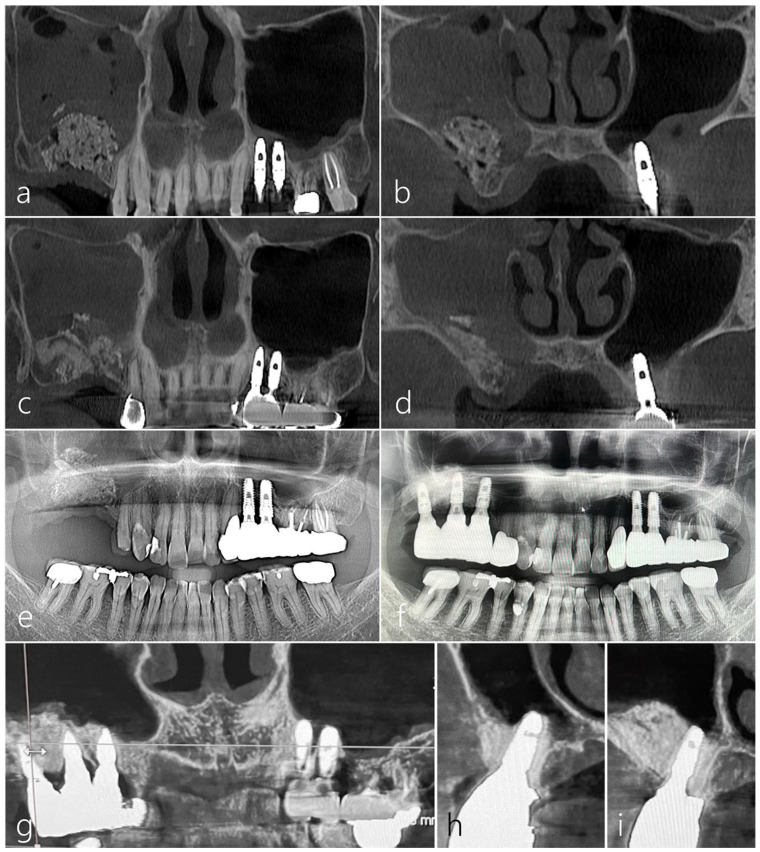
(a) The panoramic image of the CBCT taken immediately after surgery revealed an augmented bone graft in the right sinus. (b) On the coronal image of the CBCT, an augmented bone graft was observed in the buccal bone defect around the OAF site. (c) The panoramic image of CBCT taken 6 months later showed well-maintained augmented bone height. (d) On the coronal image of the CBCT taken 6 months later, some contraction of the bone graft area was observed, and the maxillary sinus was completely opacified. (e) Panoramic radiography conducted 6 months after bone grafting showed well-maintained augmented bone. (f) In panoramic radiography conducted 12 months after the prosthesis was delivered, sufficient bone graft was observed around the implants. (g) The CBCT images taken 12 months after the prosthesis delivery showed significantly reduced mucosal thickening on the right maxillary sinus. (h,i) On the CBCT images taken 12 months after the prosthesis was delivered, a well-maintained augmented bone around the implants was shown.
